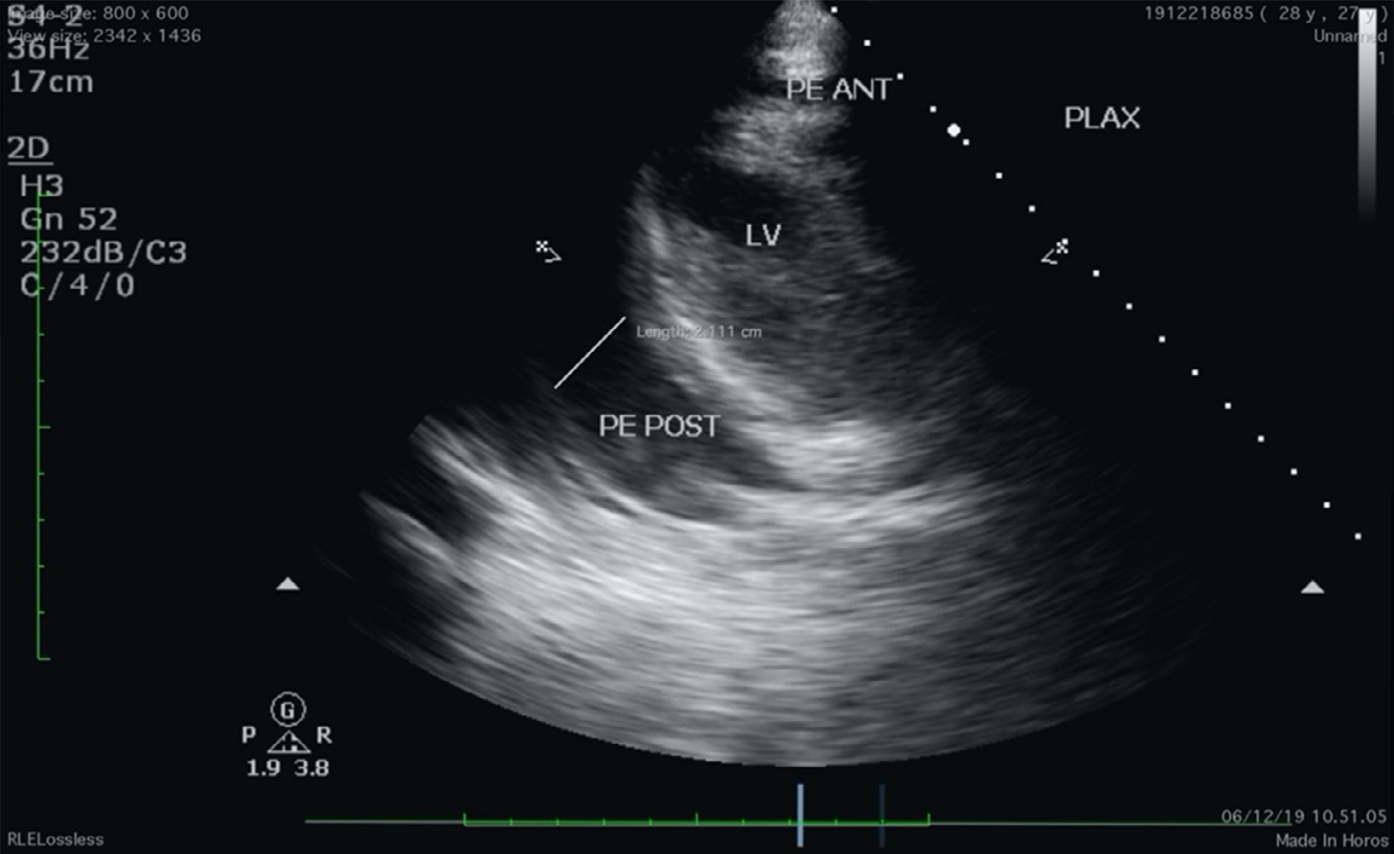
Transthoracic echocardiography demonstrates a massive pericardial effusion (21 mm) at the posterior, without any sign of right ventricle chamber collapse.
